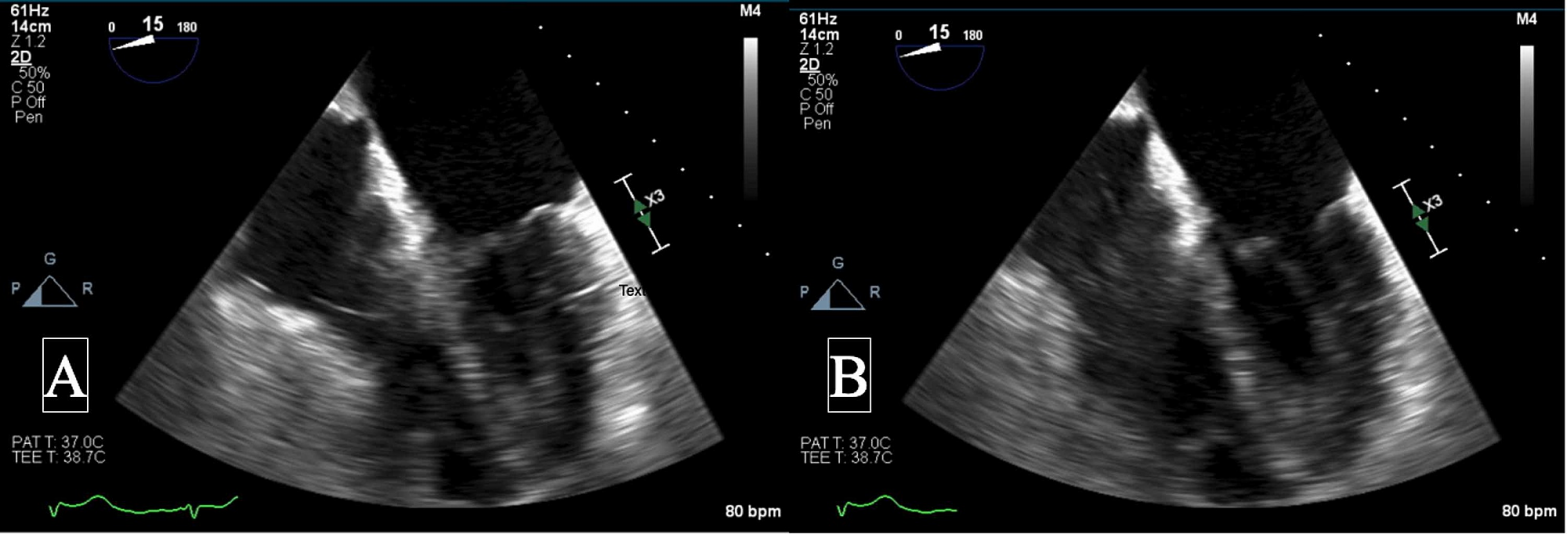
TEE: mid-esophageal four-chamber view Images are showing left ventricle (LV) systolic function recovery but persistent right ventricle (RV) apical akinesia in systolic phase (A) and diastolic phase (B). TEE - transthoracic echocardiogram
